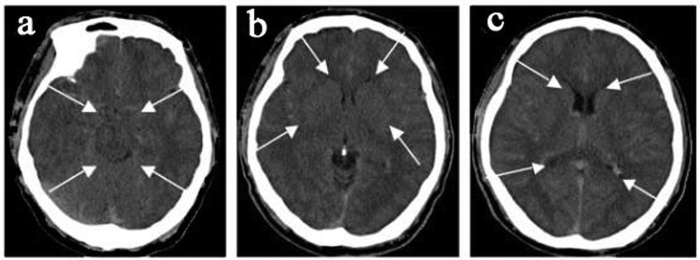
CMS patient, a 46-year-old male, hemoglobin 24.1 g/dl. CT shows diffuse cerebral edema with small ventricles (c), effaced sulci (b,c), and compromised cisterns (a,b).
